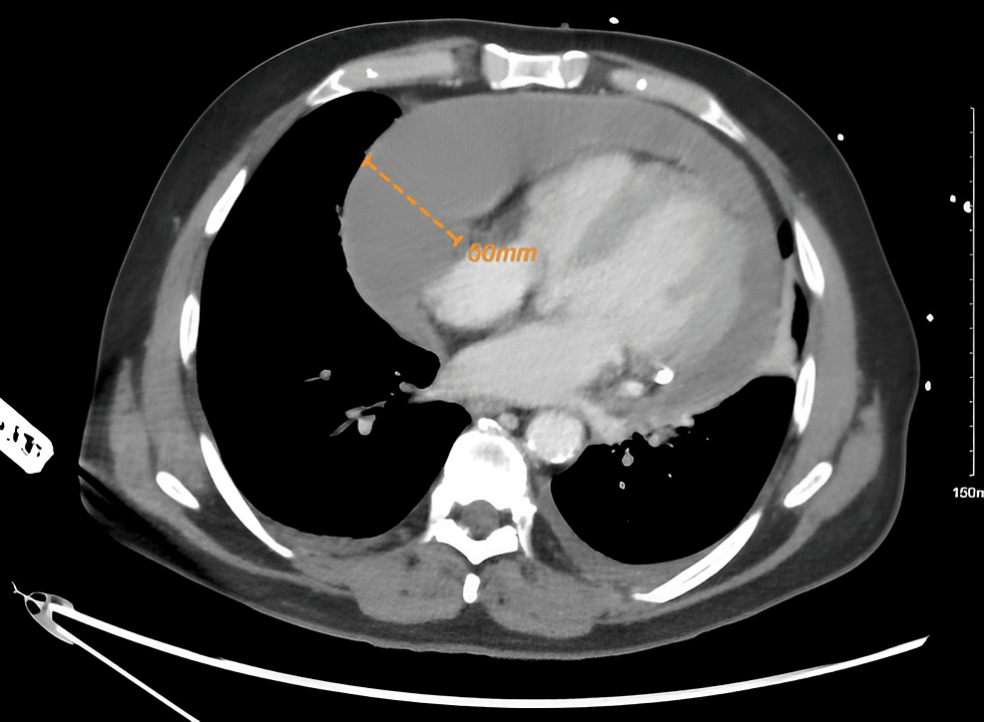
CT chest shows a large circumferential pericardial effusion measured up to 5 cm. CT: computed tomography
